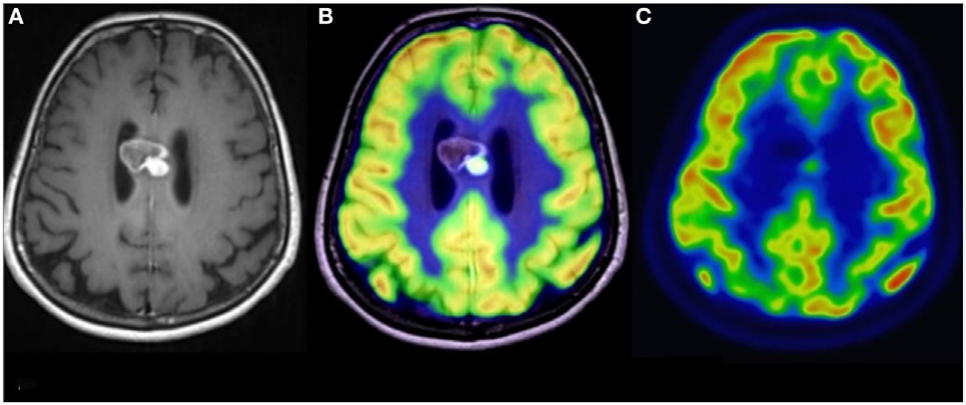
Simultaneous fluoro-2-deoxy-d-glucose (FDG) positron emission tomography (PET)-MRI of a glioblastoma. (A) Contrast-enhanced axial MR image demonstrating a heterogeneously enhancing mass in the corpus callosum. (B) Co-registered PET-MR image accurately demonstrating that the focus of elevated FDG avidity corresponds to the solidly enhancing component. (C) FDG PET image, without co-registration with MRI, demonstrates a focus of elevated FDG-avidity. No copyright permissions were required for use of these images.
